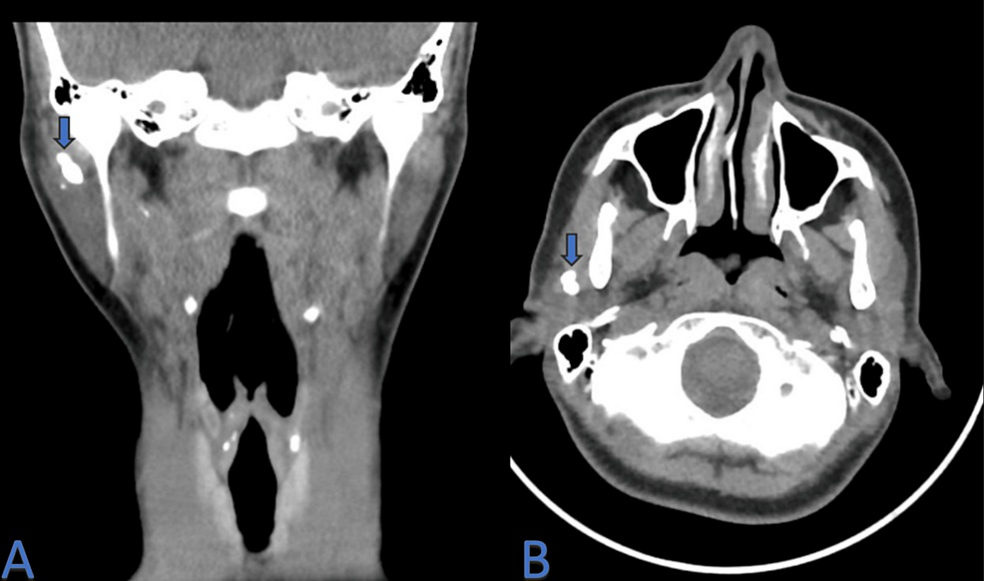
Non-contrasted neck CT scan Arrows on coronal (A) and axial (B) views of a non-contrasted neck CT scan pinpoint multiple intrasubstance radiopaque stones in the right parotid gland, with the largest measuring approximately 0.6 x 0.5 cm. CT: computed tomography
